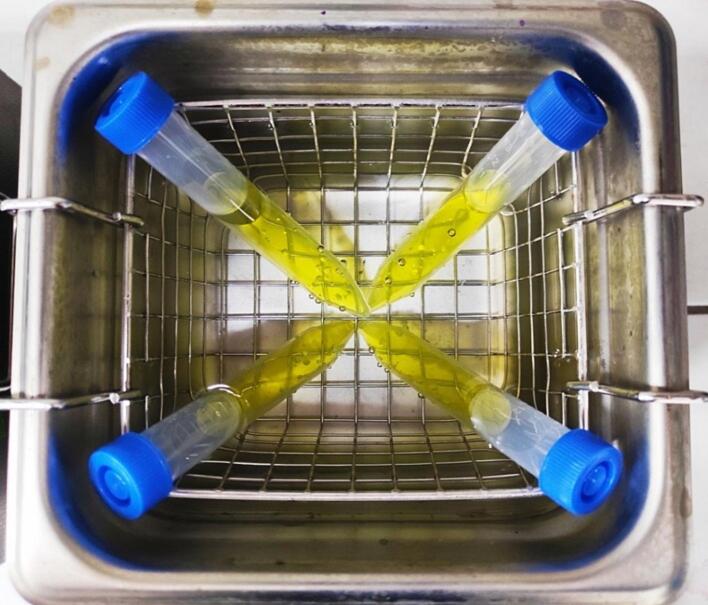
The placement of tubes in an ultrasonic bath in the sonocatalytic degradation of chromates.
